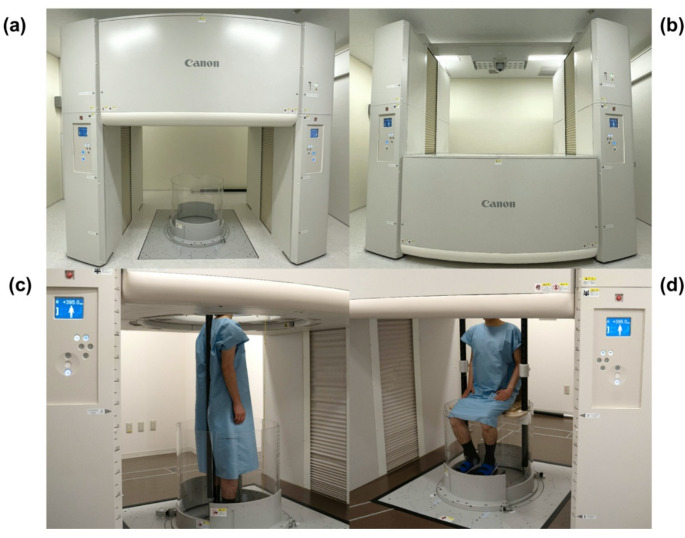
Upright multidetector CT (MDCT) system and safety-support devices. The upright MDCT system enables vertical movement of a transverse 320-detector-row gantry to perform imaging in multiple upright postures. a Gantry positioned at the upper end. b Gantry positioned at the lower end. c Standing-position imaging setup showing the support pole that provides gentle posterior stabilization and the knee-height transparent acrylic barrier that prevents falls. d Sitting-position imaging setup
